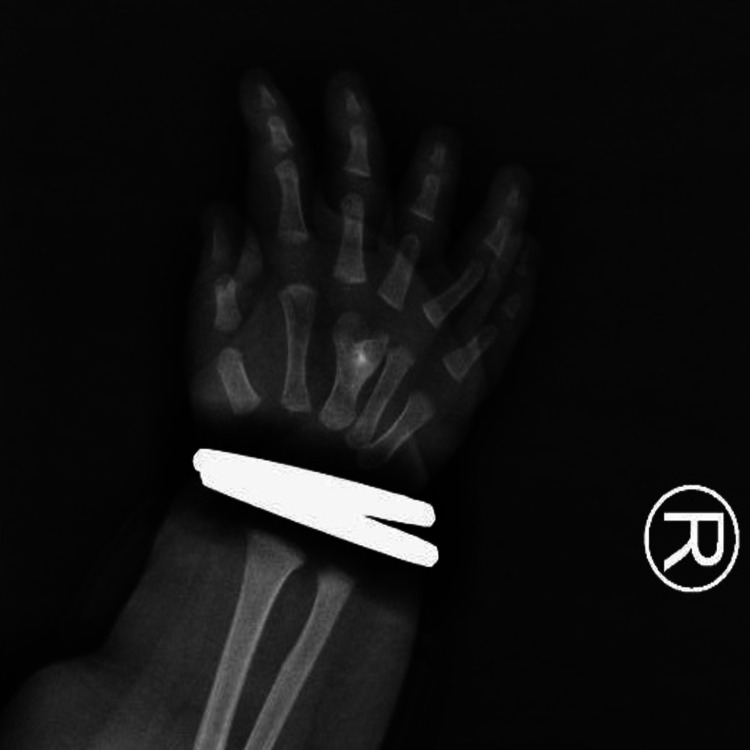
X-rays of the patient’s right hand showing an extra phalanx centrally with a bifid third metacarpal bone
